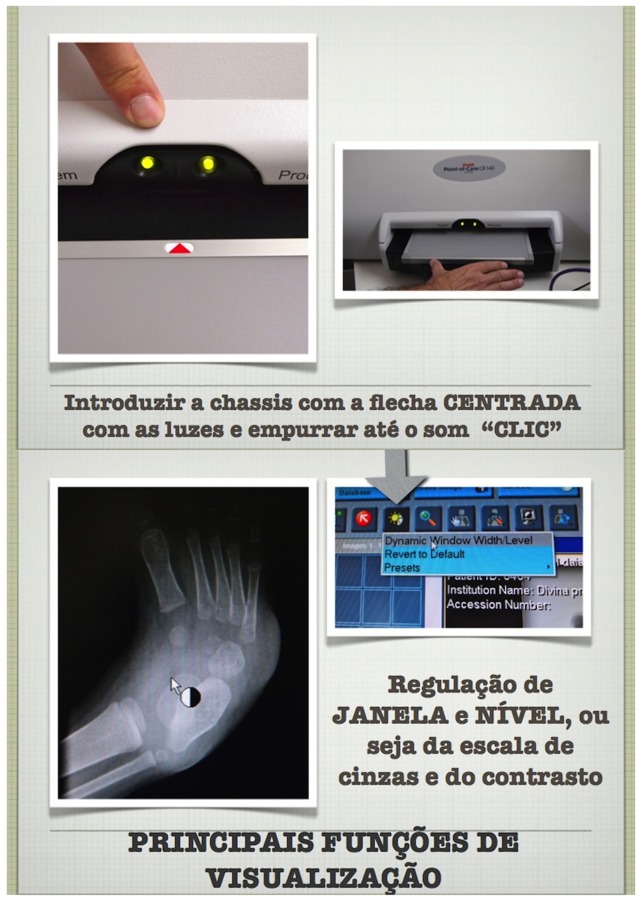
Extract from the training course. Note: This extract from the training course in Portuguese, the national language in Angola, shows how to initiate the system (upper images), and how to choose functions to optimise X-ray visualisation (lower images).
